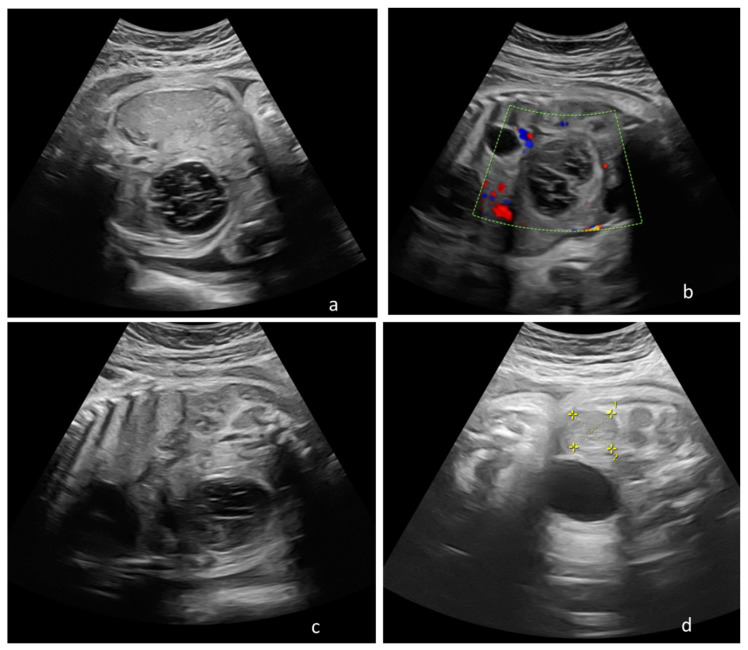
Consecutive prenatal ultrasonography depicting transformations of a fetal ovarian cyst that presented postnatal resorption, discovered at 31 weeks of gestation, and follow up until 36 weeks of gestation in a woman diagnosed with severe preeclampsia. (a) Thirty-one weeks of gestation female fetus with an 3 cm diameter echogenic right ovarian cyst with a thing wall, suggesting intracystic hemorrhage; (b) 32 weeks and 4 days, the changes of the cyst are followed, which becomes elongated, keeping the appearance of a intracystic hemorrhage, measuring 4.5/4 cm; (c) same case at 33 weeks and four days the fetal cystic begins to clarify its contents and maintains its decreases it diameter at 4.5/3.5 cm (d) same case at 36 weeks and four days, the fetal cyst is in important resorption measuring 2.32/2.39 cm. Figure 3a,c,d represent B-mode ultrasound images, while Figure 3b is CFM (Colour Flow Mapping) ultrasound mode that shows peripheral blood flow.
